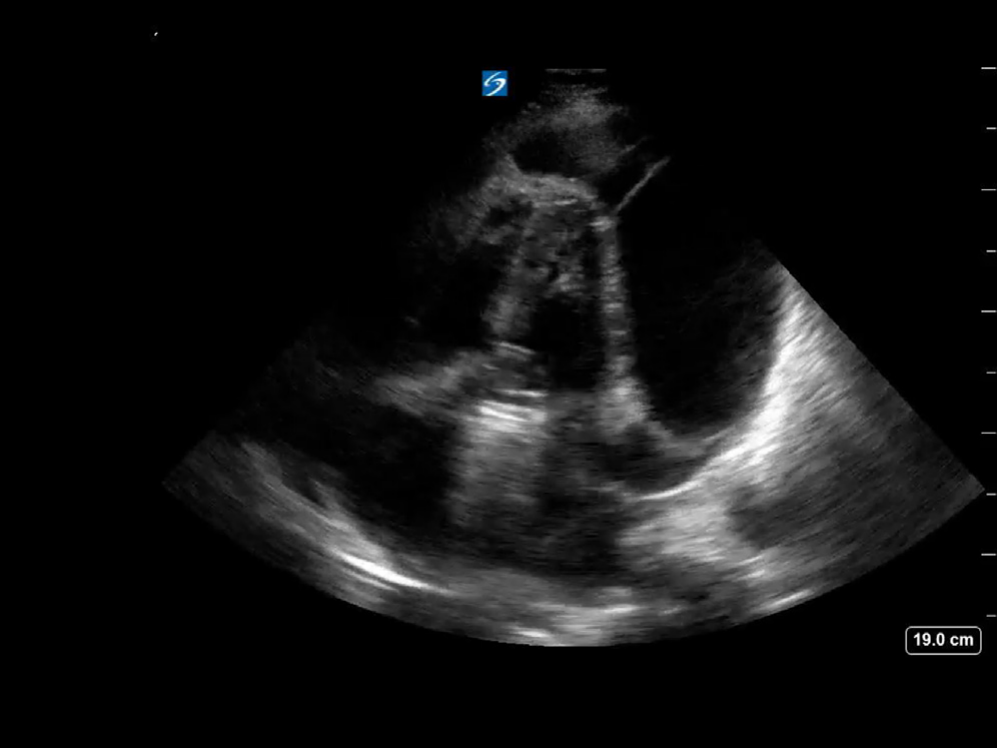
Apical 4 chamber view of the heart demonstrating a large circumferential complex pericardial effusion. Fibrin stranding is demonstrated beating with the atrioventricular valves. Signs of tamponade physiology are seen with both right ventricular and left ventricular collapse in diastole.
